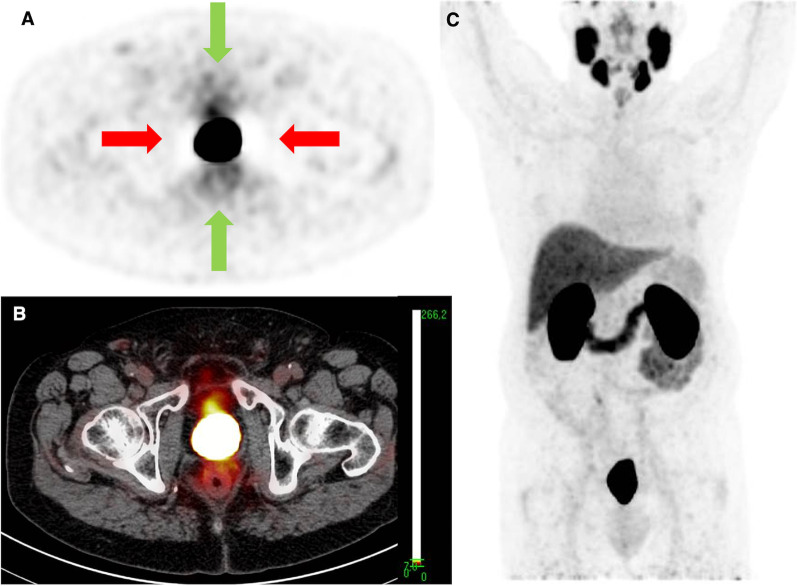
PSMA PET/CT with ^18^F-DCFPyL used as tracer in a 68 years old man staged for staging of biochemical recurrence of prostate cancer showing peri-bladder artefacts, deteriorating image quality and impairing image interpretation. A Axial PET reconstructions; B fused axial PET/CT reconstructions; and C PET maximum intensity projection (MIP). Window width SUV range 0–7 applied. Red arrows: PET photopenic area (halo artefact). Green arrows: increased PET activity (flare artefact).
